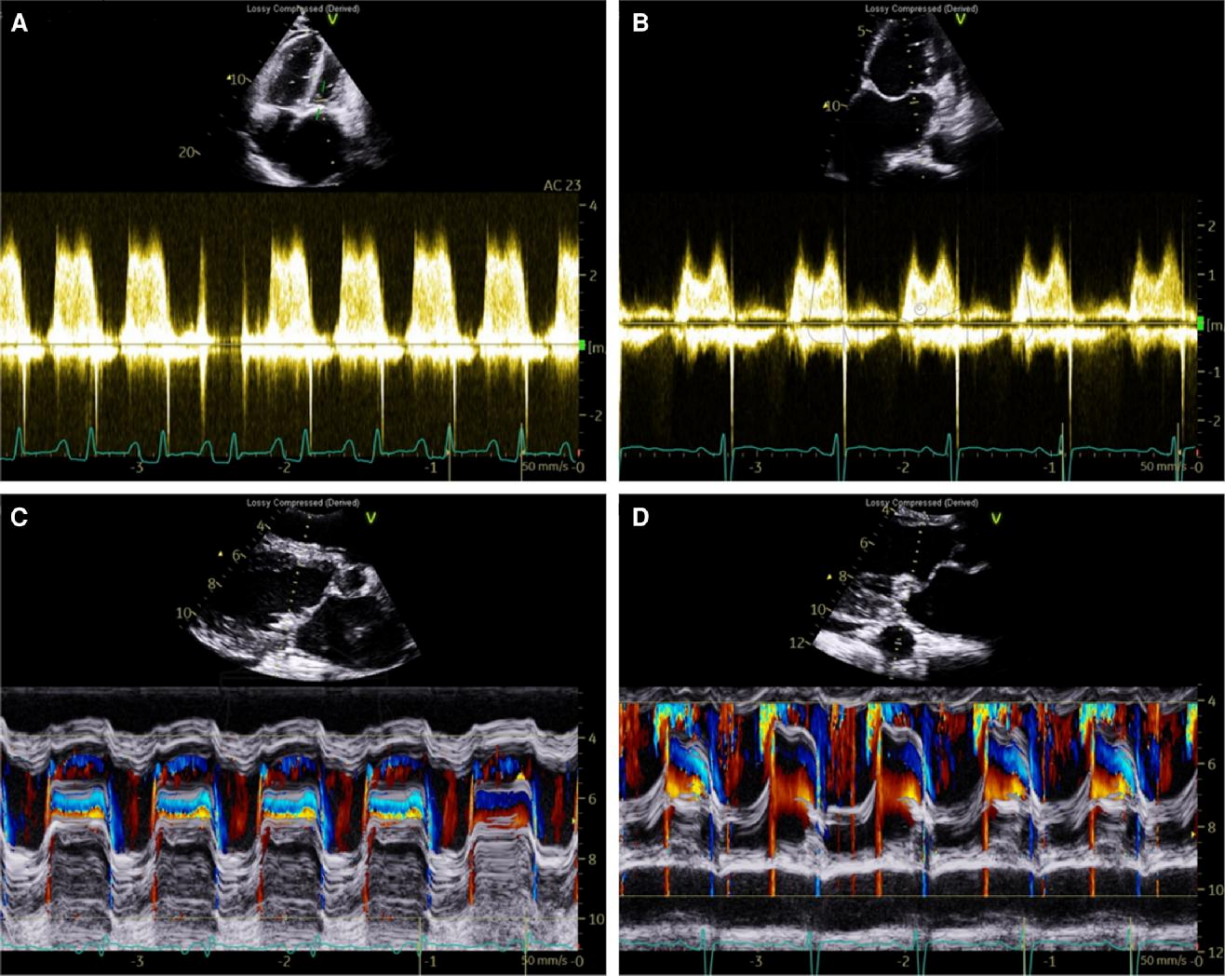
(*A*) Doppler wave transthoracic echocardiogram (TTE) pre-percutaneous transcatheter mitral commissurotomy (PTMC) showing severe mitral valve (MV) stenosis with high mean diastolic gradient and left atrial (LA) dilatation. (*B*) Post-PTMC TTE demonstrating significant improvement in the MV mean diastolic gradient. (*C*) M-mode parasternal long axis (PLAX) pre-PTMC showing severe MV stenosis with restricted movement and opening between the anterior and posterior leaflets and LA dilatation. (*D*) Post-PTMC M-mode PLAX demonstrating significant improvement in the leaflet’s movement and release of the restriction.
